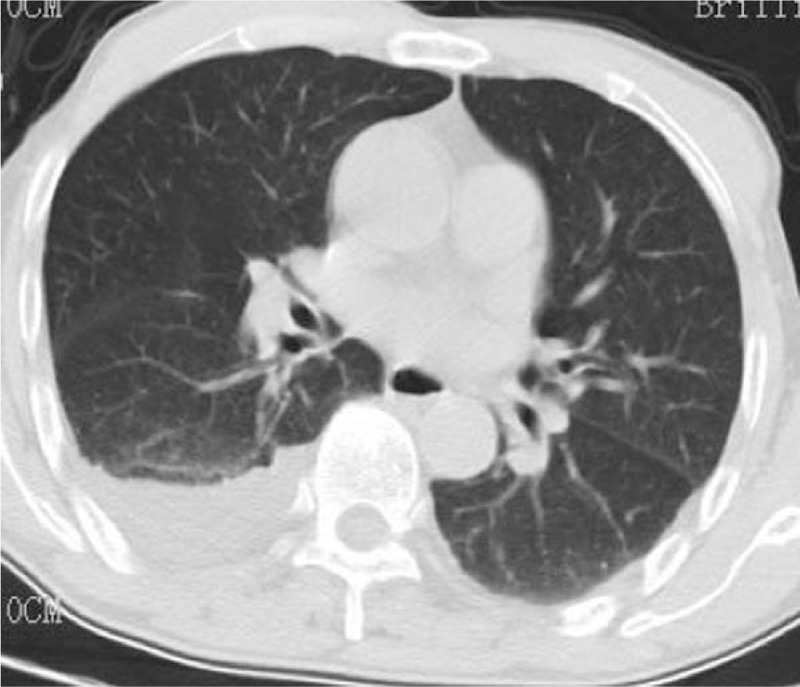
Chest computed tomography scan at presentation, showing a bilateral and massive left-side pleural effusion.
